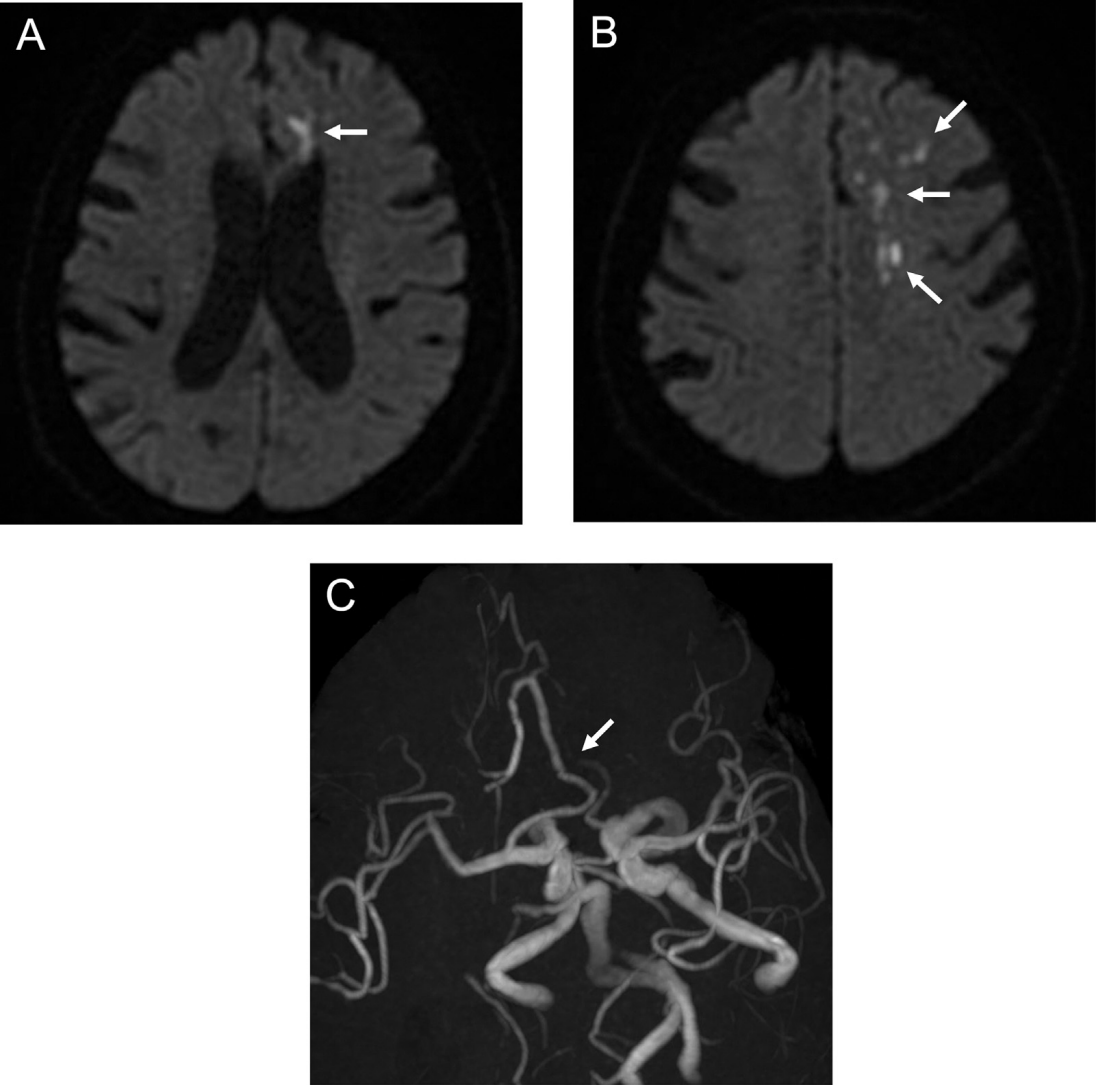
Preoperative magnetic resonance imaging and magnetic resonance angiography. Diffusion-weighted imaging showing multiple fresh infarcts (arrows) (A, B). Magnetic resonance angiography showing poor delineation of the left anterior cerebral artery, especially distal to the A2 segment (arrow) (C).
